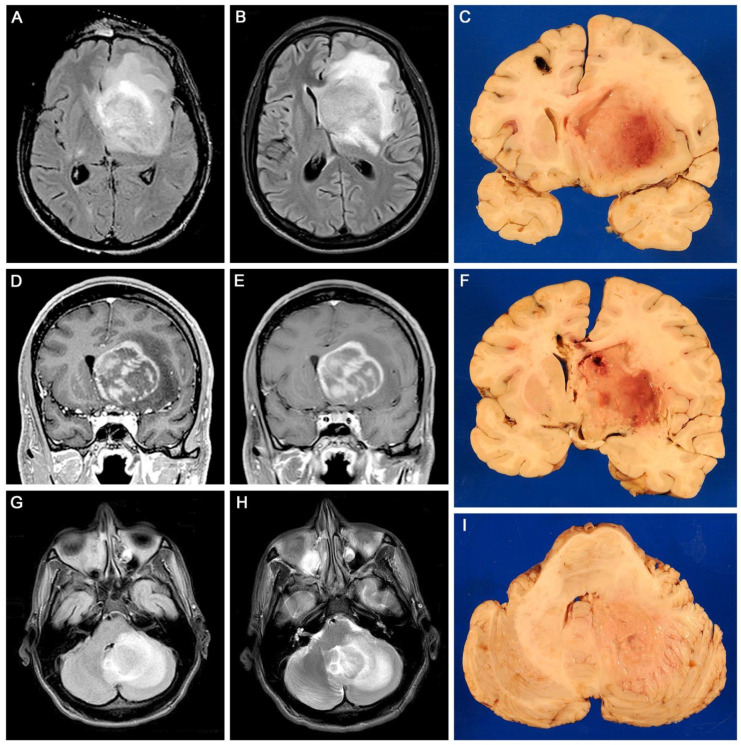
Neuroimaging and neuropathology: Axial plane MRI shows a large mass in the right basal ganglia which causes significant edema (Panels (A,B)). Coronal MRI shows the mas is ring enhancing (Panels (D,E)). Coronal sections of the brain corroborated a large mass of poorly defined edges with prominent areas of necrosis, located in the right basal ganglia with areas of necrosis, that causes significant edema and compresses the lateral ventricle; the site of the surgical probe can be seen on the contralateral frontal lobe (Panels (A–F)). Another similar homogeneous mass can be seen in the right cerebellar hemisphere, causing a prominent midline shift (Panels (G–I)).
